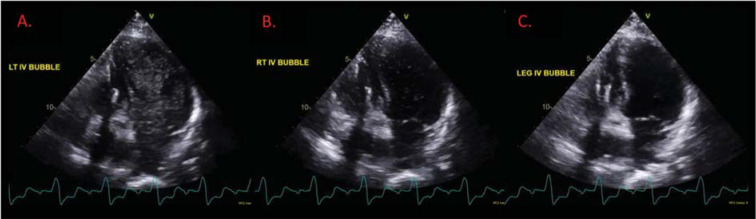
Follow-up transthoracic echocardiogram with agitated saline contrast injected from bilateral upper extremities and through the lower extremity to reassess shunting after coil embolization of a large collateral. A: A large number of agitated saline bubbles appeared in the left atrium and left ventricle before the right side with the left-sided injection in a manner suggestive of persistent extracardiac shunting. B: With the right upper-extremity injection, the density of the bubbles was reduced, following the same route in a manner suggestive of a lesser degree of shunt on the right compared to the left. C: An absence of agitated saline bubbles was seen with the lower-extremity injection through multiple cardiac cycles. *Abbreviations:* IV, intravenous; LT, left; RT, right; TTE, transthoracic echocardiogram.
